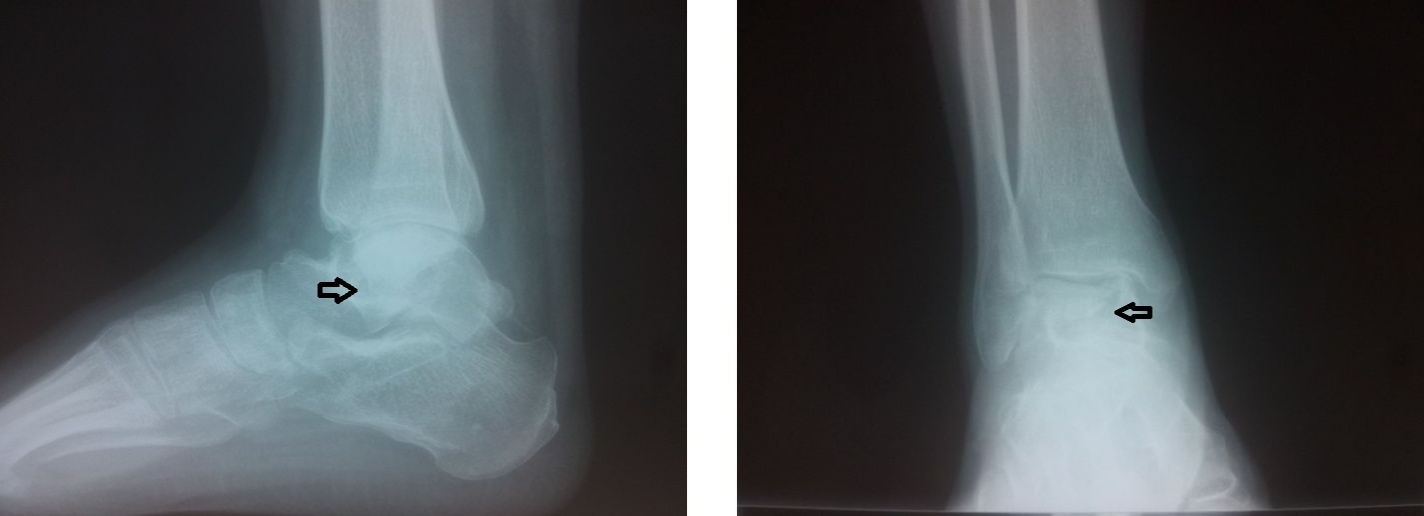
Plain radiographs of the right ankle's anteroposterior and lateral views. Extensive lytic lesion in the body of talus (black arrows).
